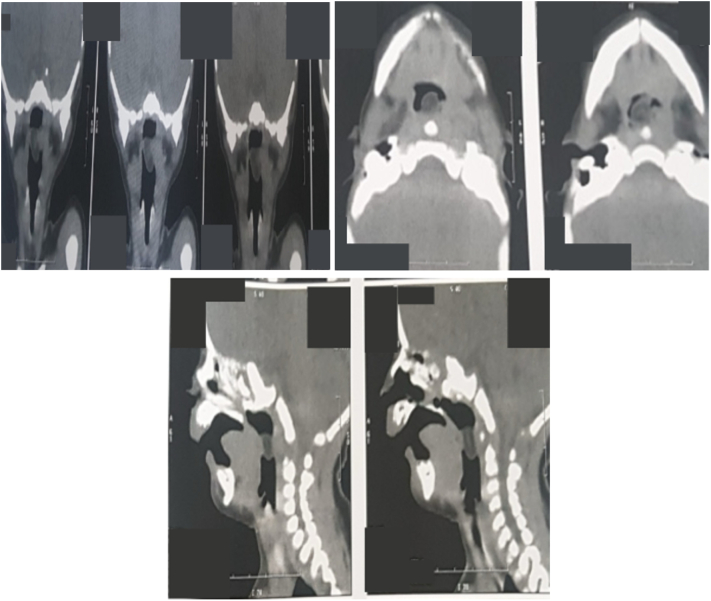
CT scan showing hamartoma of the soft palate as a mass of mixed tissue and fat density measuring 5.9 × 6.6 × 13.7 mm, filling the lumen in front of and respecting the valleculas, base of tongue and prevertebral space.
